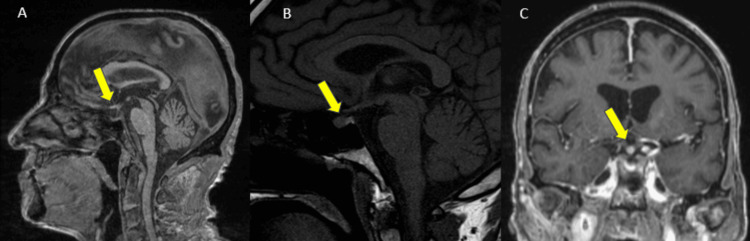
Sagittal T1 MRI demonstrates lack of pituitary bright spot (A), compared to a normal patient (B, yellow arrows). Coronal T1FS Gd (C) also shows focal nodular enhancement of the pituitary stalk (yellow arrow) MRI: magnetic resonance imaging; T1FS Gd: T1 fat-saturated post-gadolinium
